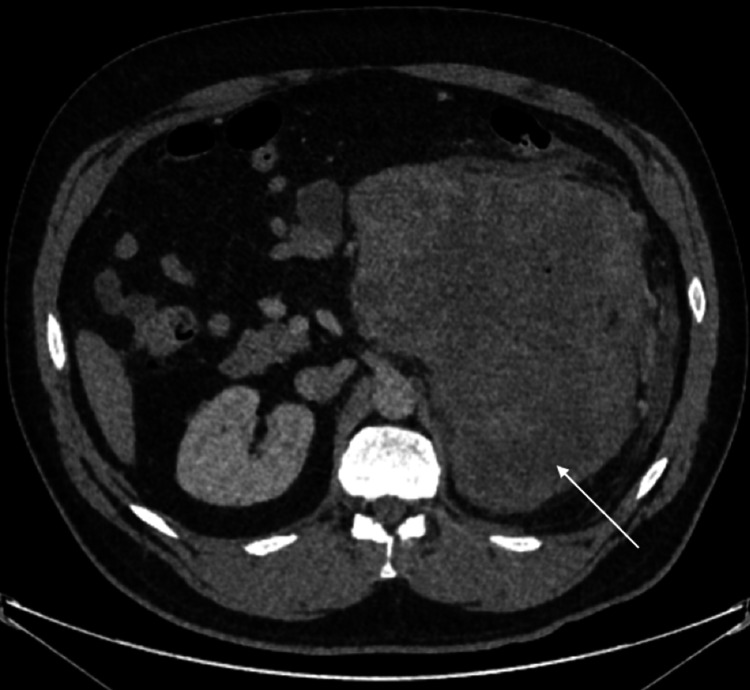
CT scan of the abdomen with contrast demonstrating large retroperitoneal mass encasing the left kidney and vessels (arrow).
